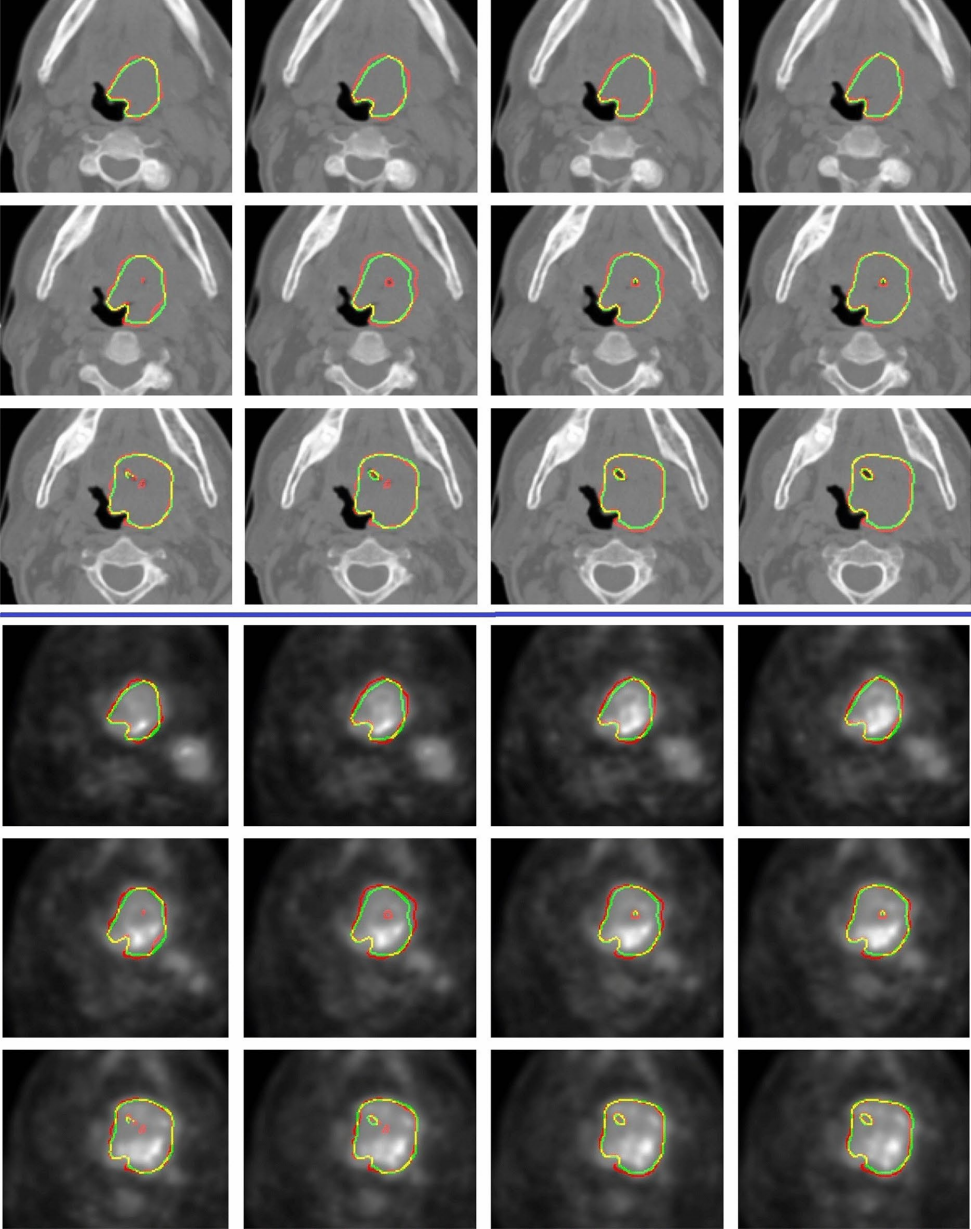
The figure depicts examples of consecutive slices from a 3D CT and PET volume, respectively, in HECKTOR dataset (CHUM center, volume 6, slice 32nd onwards.). The original tumor boundary is highlighted in red, while the predicted boundary is highlighted in green. The intersection of the original and predicted boundaries is shown in yellow.
